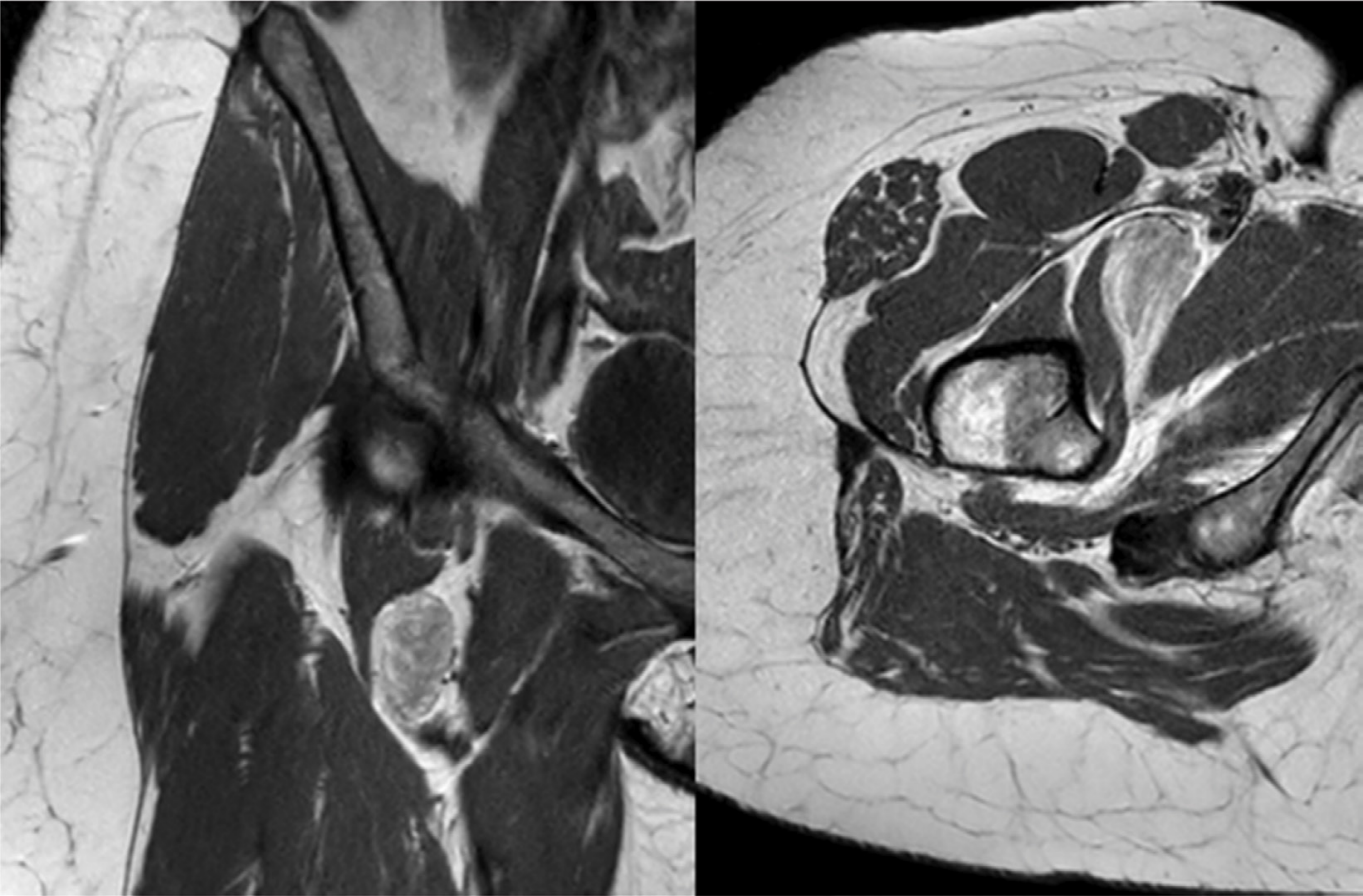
Coronal and axial T1 weighted MRI images revealed a well-circumscribed mass in the deep right inguinal region with mixed signal intensities.
